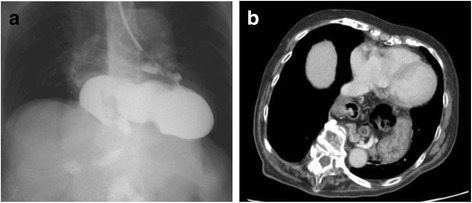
Giant hiatal hernia. a An upper gastrointestinal contrast study showed an upside-down stomach. b Computed tomography revealed a giant hiatal hernia with the intrathoracic stomach located behind the heart and compressing the left atrium
